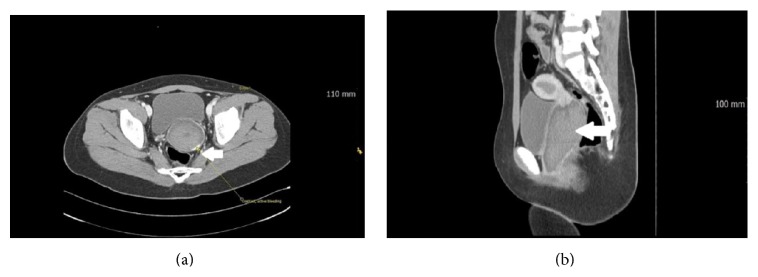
Computed tomographic findings. (a) Axial view. Arrow shows contrast extravasation and active bleeding in vagina. (b) Blood-filled vagina means hematocolpos in sagittal view.
